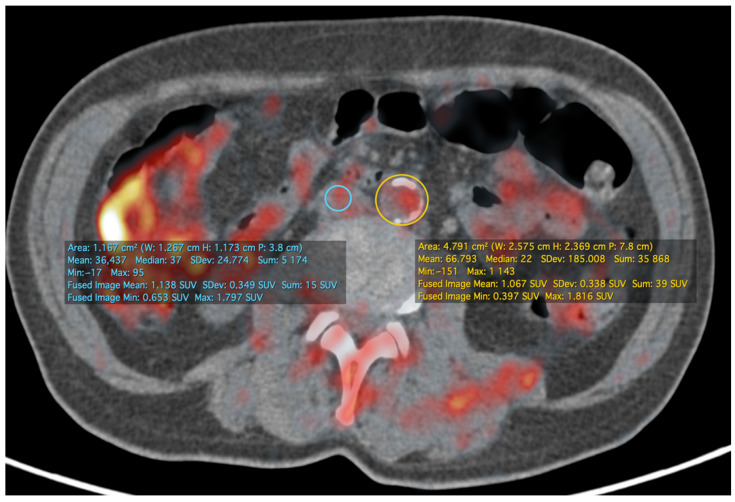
The same patient and study as in Figure 1. At the infrarenal level of the aorta, more prominent calcific atherosclerotic plaques are visible. The image shows a method of calculating TBR as a ratio of SUV in the aorta region of interest and SUV in the venous blood pool on the same level—in this case, the inferior vena cava was used. TBRmax at this level was about 1.01. All calculations were performed in OsiriX MD (Pixmeo SARL, Bernex, Switzerland).
